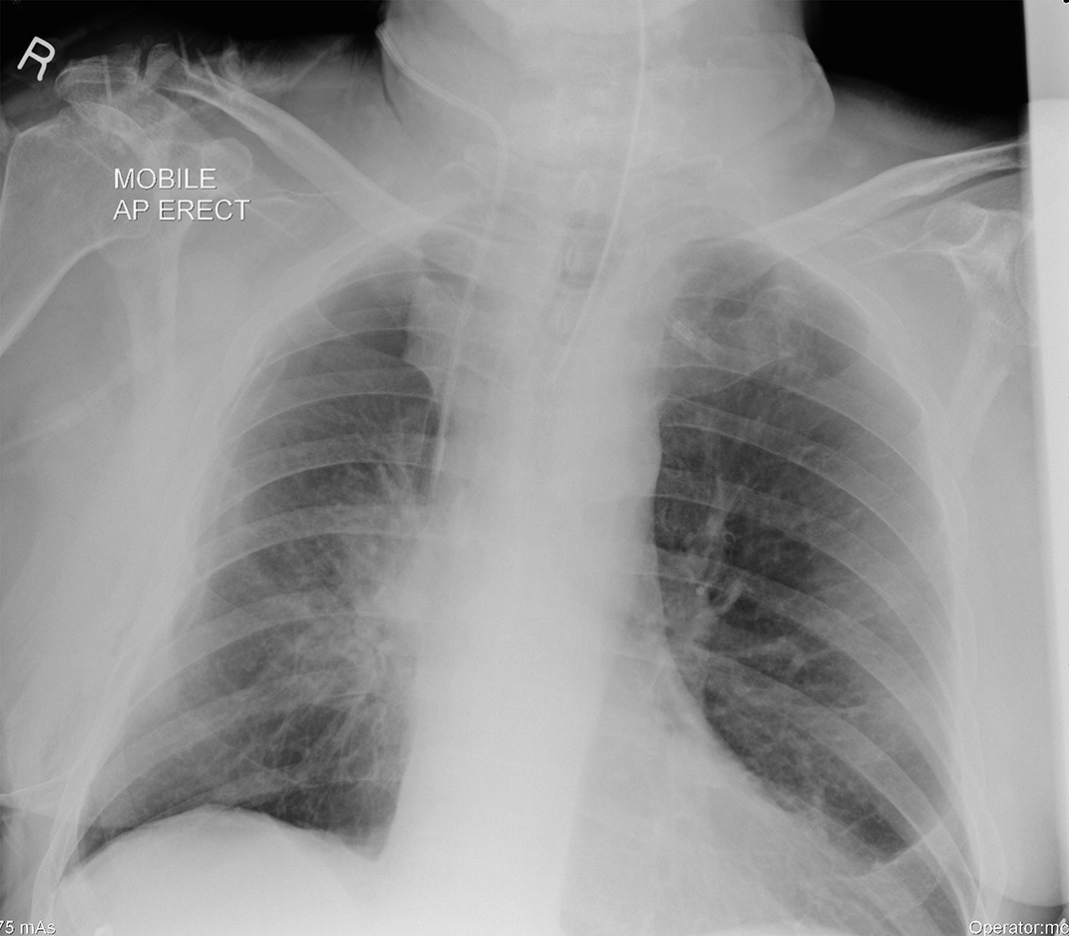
The first post-operative chest radiograph performed within hours of upper lobectomy. There is increased density adjacent to the right hilum but no lobar parenchymal consolidation. A right central venous catheter, endotracheal tube and right chest drain are also demonstrated. AP, anteroposterior.
